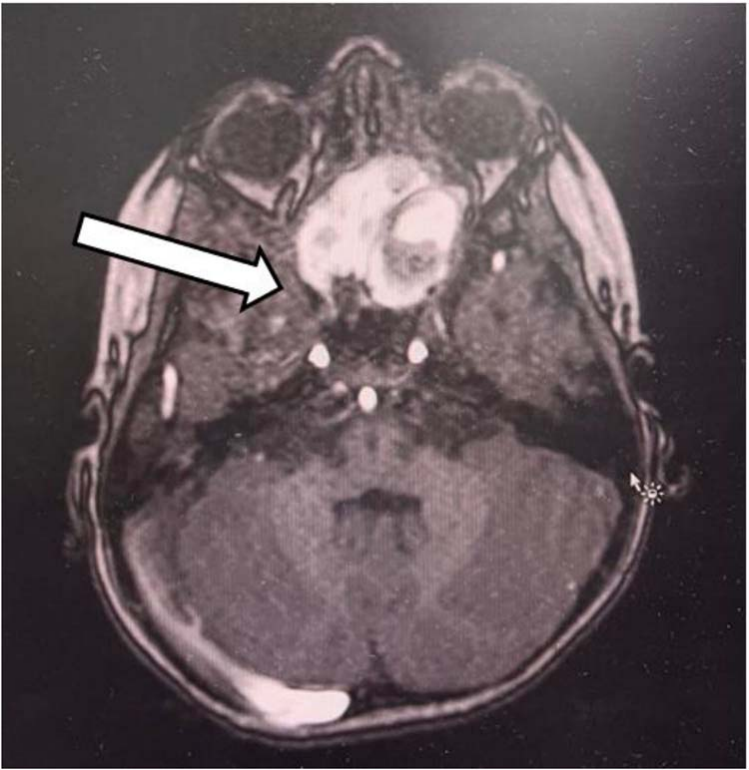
The axial section of the MRI.
